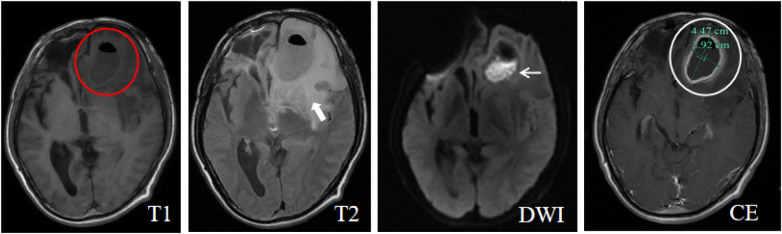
Contrast-enhanced cranial MRI: A cystic space-occupying lesion is visualized on the T1-weighted sequence, with the density of the cyst fluid higher than that of cerebrospinal fluid and gas present inside the cyst (red circle); extensive cerebral edema is observed around the cystic space-occupying lesion on the T2-weighted sequence (thick arrow); the cyst fluid shows hyperintensity changes on the DWI sequence (thin arrow); and ring enhancement of the cyst wall of the space-occupying lesion is seen on the contrast-enhanced sequence, with a size of 4.47 cm × 2.92 cm(white circle).
